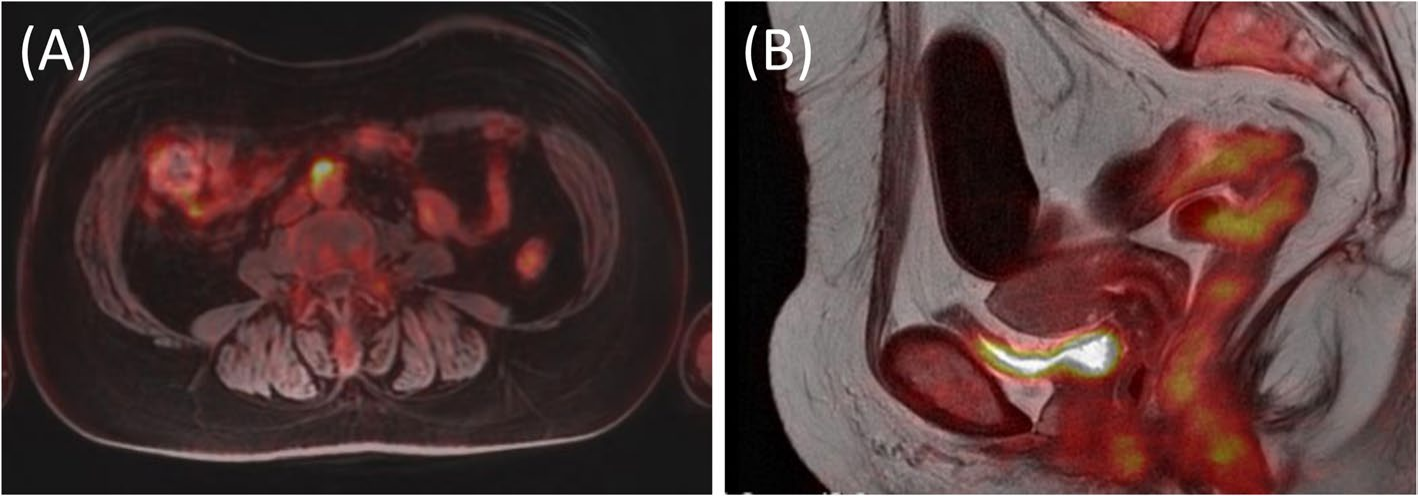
A On a PET-MRI, enlarged paraaortic lymph nodes with FDG accumulation were detected. B No obvious mass lesion was observed in the uterus
